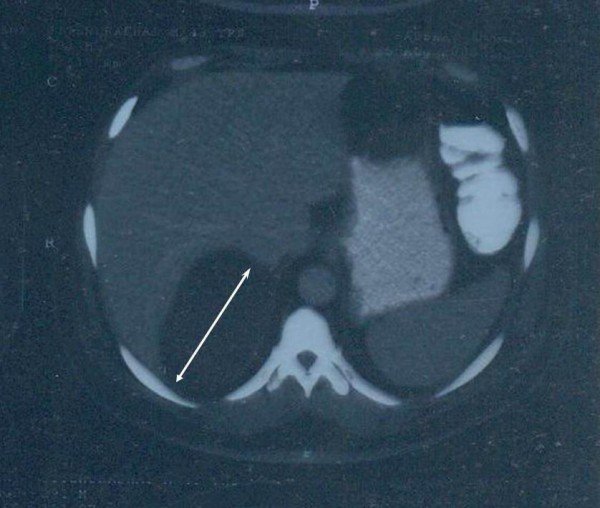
Computed tomography scan showing a large, well-defined, soft tissue density mass in the right suprarenal region (bidirectional arrow).
